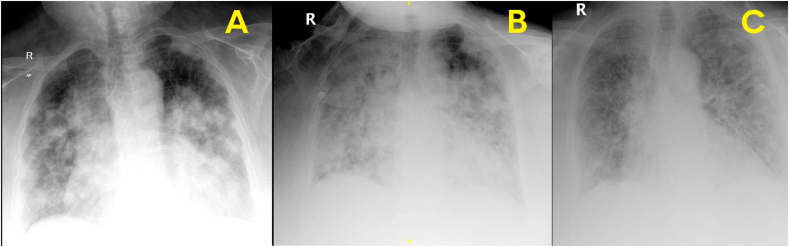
A) Admission Chest X-Ray; B) Day 10 Chest X-Ray; C) Discharge chest X-Ray.
